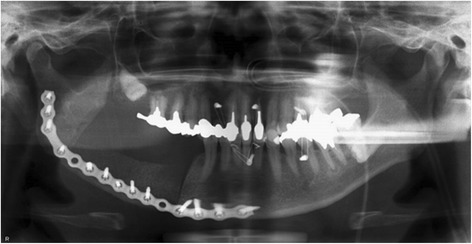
Postoperative panoramic radiograph in case 1. Both the occlusion and position of condylar heads in the temporomandibular fossae were restored to the preoperative position
